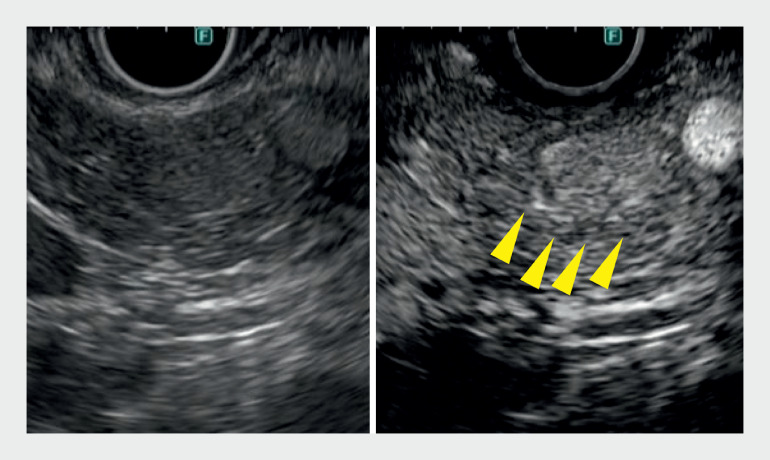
Contrast-enhanced endoscopic ultrasound depicted a mildly hypervascularized tumor (arrows), although the boundary with the surrounding area was somewhat difficult to discern.
